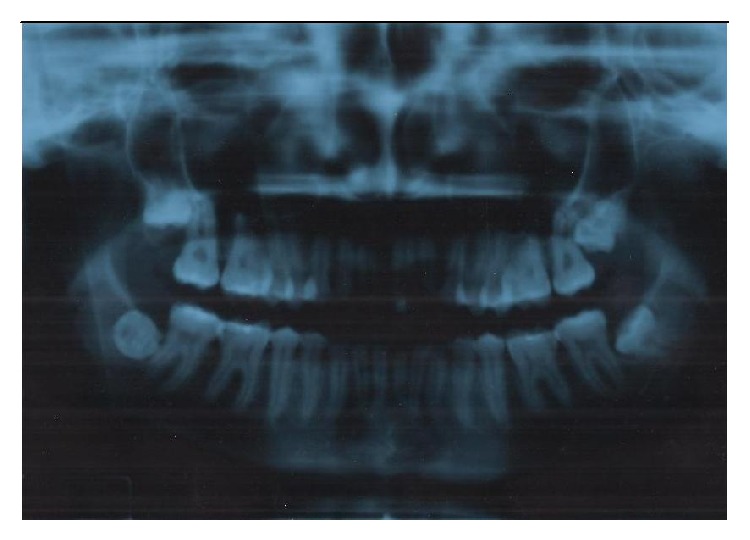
X-ray before any intervention.
